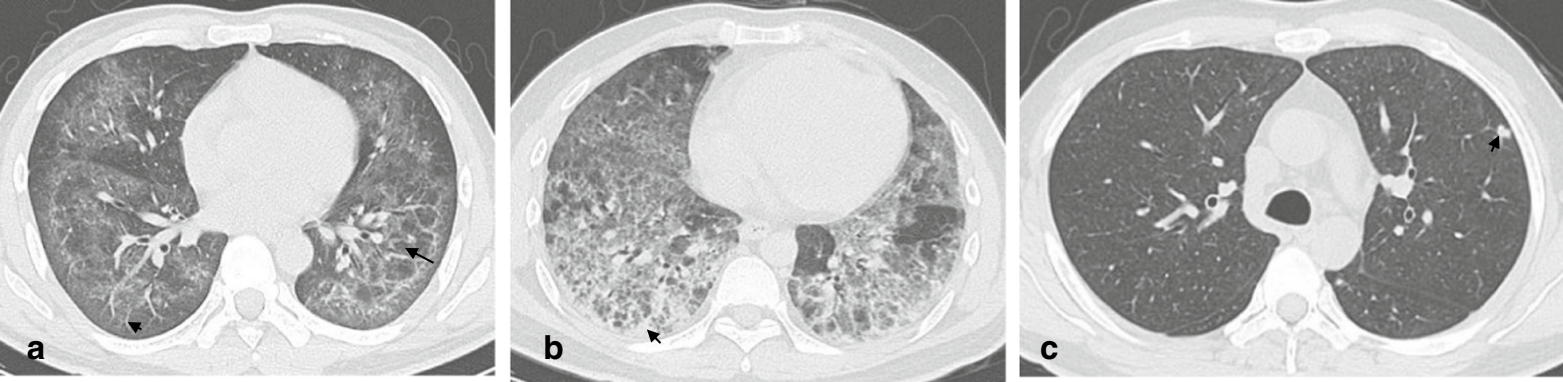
Cytomegalovirus pneumonia. a Pneumonia caused by cytomegalovirus in a 38-year-old male patient with AIDS. HRCT image showed ground-glass opacity, reticulation (arrow), and mosaic perfusion (long arrow). b Pneumonia caused by cytomegalovirus in a 36-year-old male patient with AIDS. HRCT image showed ground-glass opacity, reticulation, consolidation (arrow), and mosaic perfusion. c Pneumonia caused by cytomegalovirus in a 29-year-old male patient with AIDS. HRCT image showed ground-glass opacity, nodules with halo sign (arrow) and tree in bud sign
